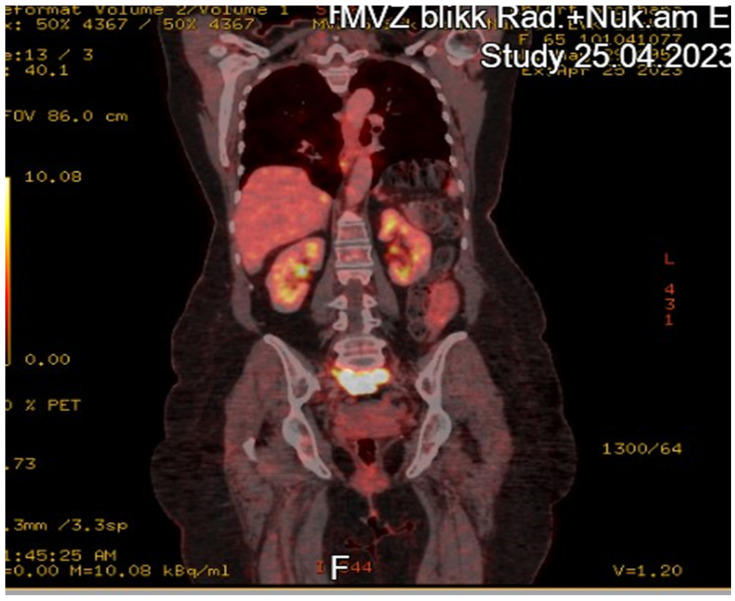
Coronal ^18F FDG PET CT demonstrating intense hyper metabolism (SUVmax = 13.2) of the presacral carcinosarcoma with encasement of the right common iliac vein.
